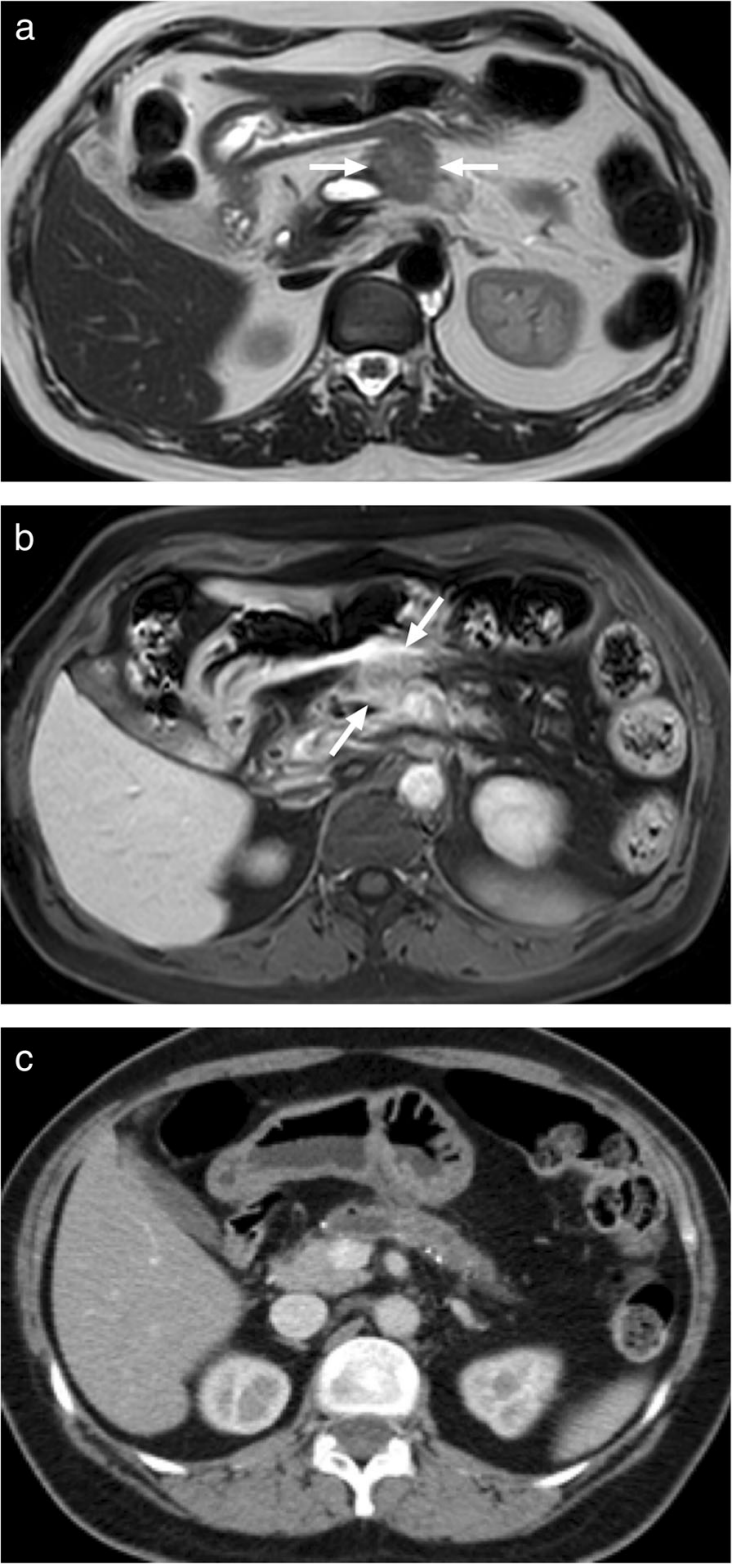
A 54-year-old female with known alcohol induced chronic pancreatitis and chronic pain now presenting with unusually intense epigastric pain. a T2W MRI demonstrates a hypointense ill-defined 3-cm-diameter solid mass (arrows) in the pancreatic body/tail with downstream ductal dilatation, likely related to patient’s known chronic pancreatitis. b Axial plane postcontrast T1W image demonstrates heterogeneous enhancement of this lesion (arrows). The patient was scheduled for surgical excision. c Two weeks after the initial MRI scan, immediately before the planned surgery, the CT scan showed that the mass had almost completely resolved without any treatment. Based on these findings, the mass was considered to represent a pseudomass due to focal pancreatitis
